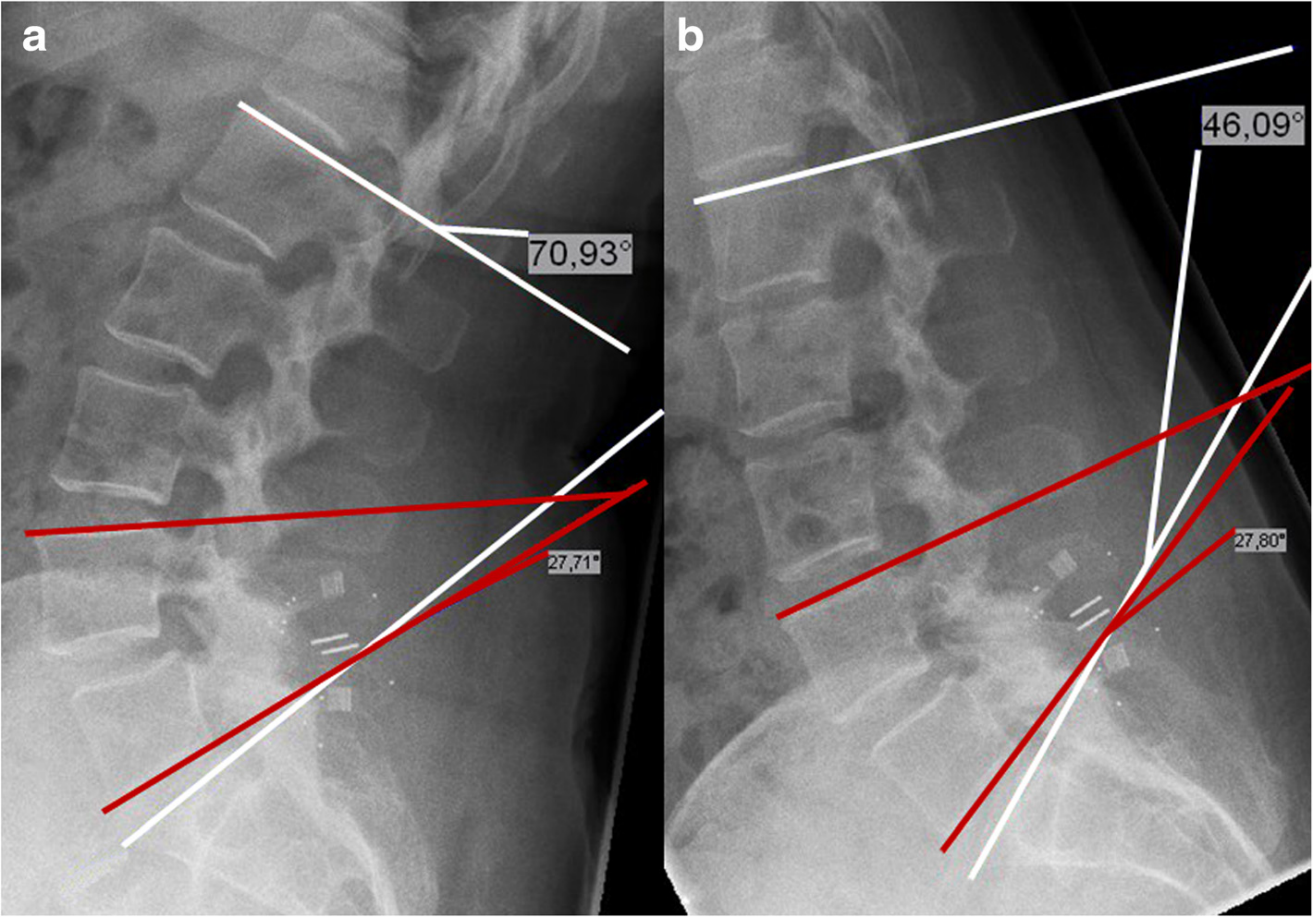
Measurement of the segmental and total lumbar angle. Measurement in the lateral roentgenogram was performed with the Cobb method with the implant fixed between the spinous processes of L4 and L5. The red lines show the segmental angle measured between the upper endplate of L4 and the lower endplate of L5, the white lines label the total lumbar angle measured between the upper endplate of L1 and the endplate of S1. a: Extension, b: Flexion
